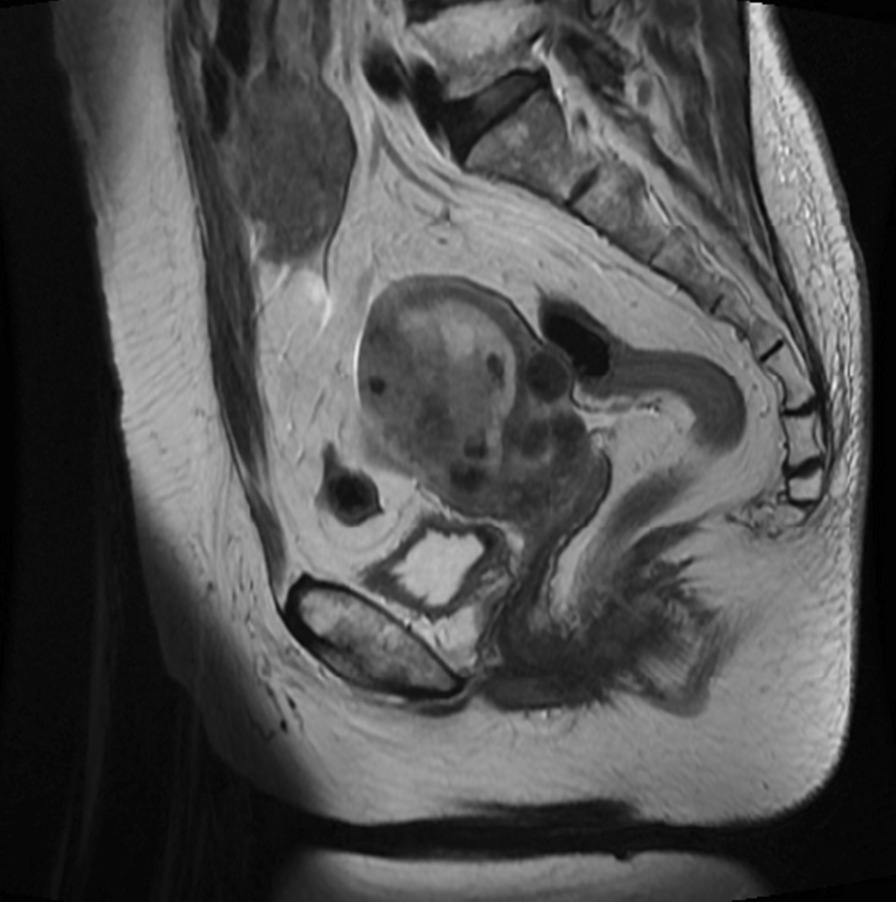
Magnetic resonance imaging of the pelvis. T2-weighted imaging reveals a thickened endometrial mass (60 × 66 × 53 mm)
